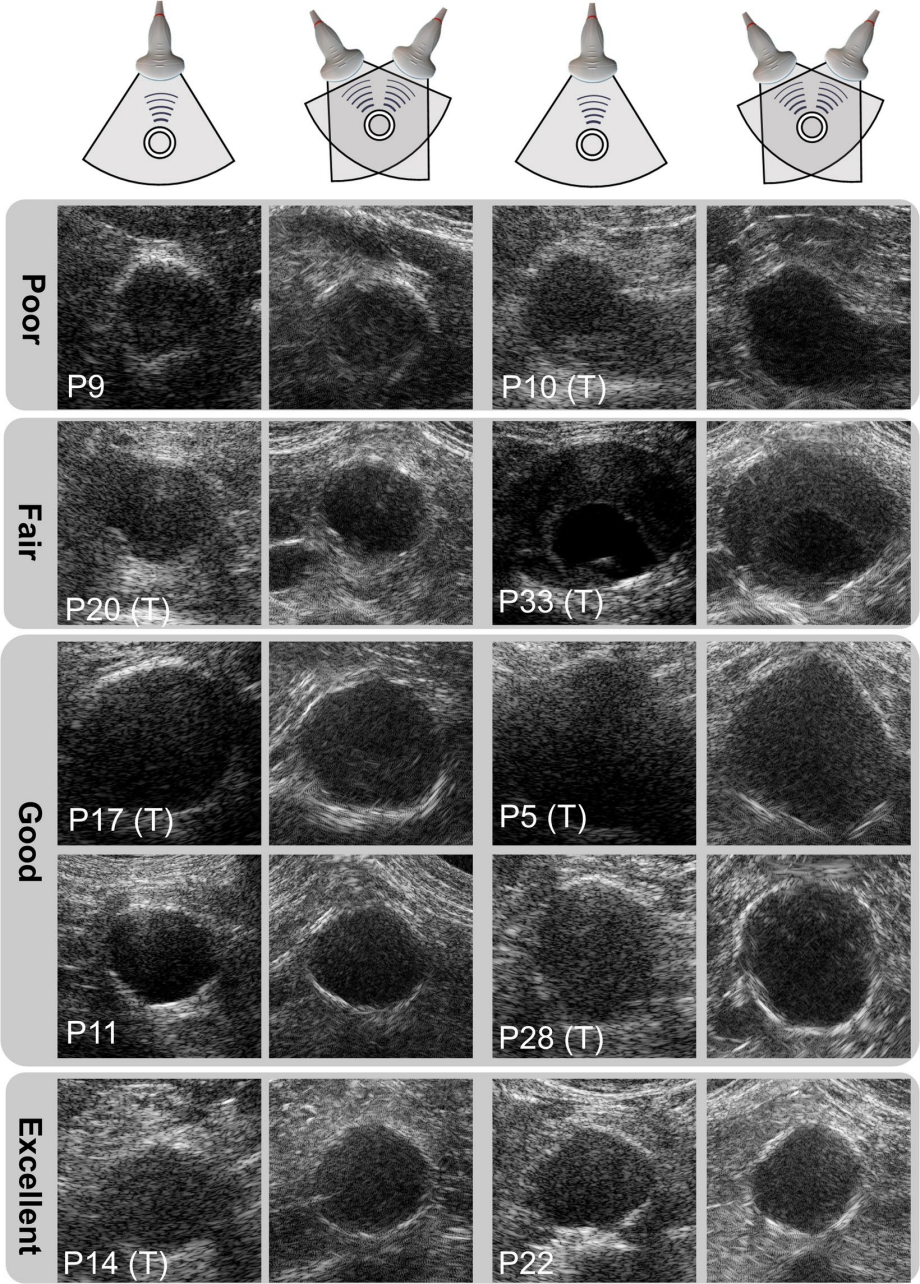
Zoomed-in ultrasound images of the abdominal aortic aneurysm using single-aperture ultrasound imaging (left) and bistatic coherent dual-aperture ultrasound imaging (right). All images have the same size of 7$$\times$$7 cm. The (T) behind the patient number indicates whether ILT is present. Images are displayed according to the average image quality score of the bistatic US images, as scored by five professionals. The dynamic range of all images is 55dB. Contrast stretching is applied with $$\alpha$$=1.3.
